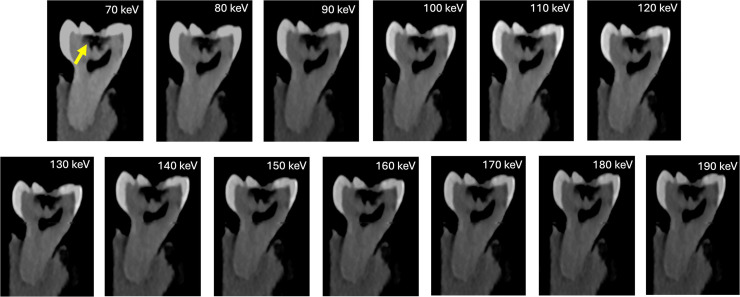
Carious lesion (arrow) depiction across PCD-CT VMI reconstructions (70-190 keV). Lesions showed clear and confident visualization at low VMI levels (70–80 keV), with a slight drop at higher levels (≥100 keV). Artifacts were minimal or absent across all VMI levels.
